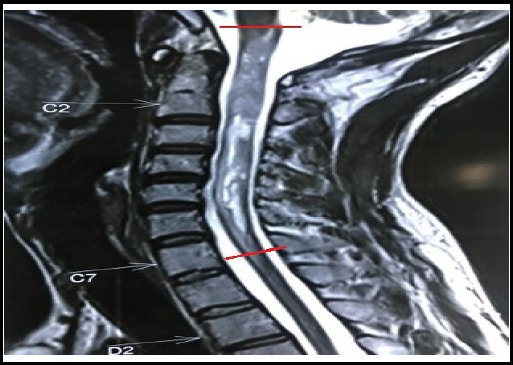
MRI T2 sequence showing intramedullary spinal tumor extending from cervico-medullary junction to C7 level.
